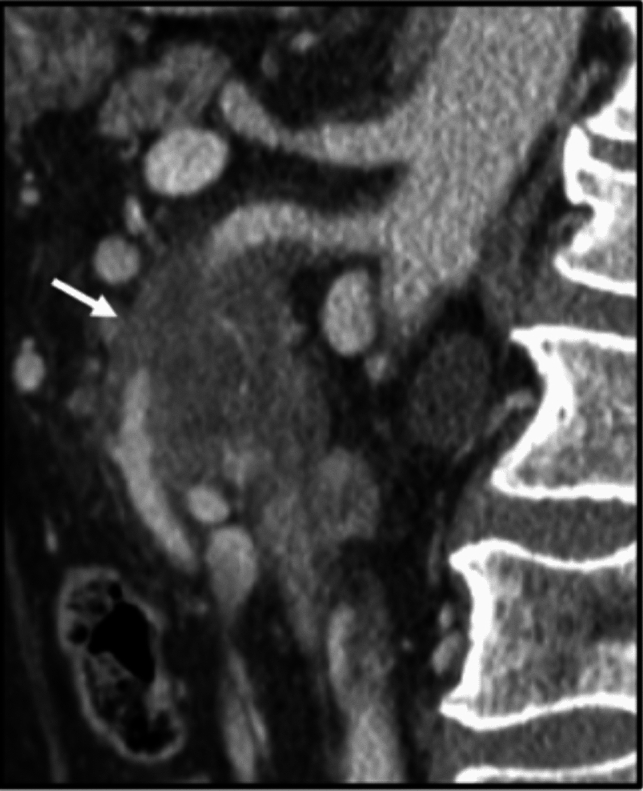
Sagittal CT section with portal venous phase contrast. Hypoenhancing tumor encases the superior mesenteric artery (arrow)
